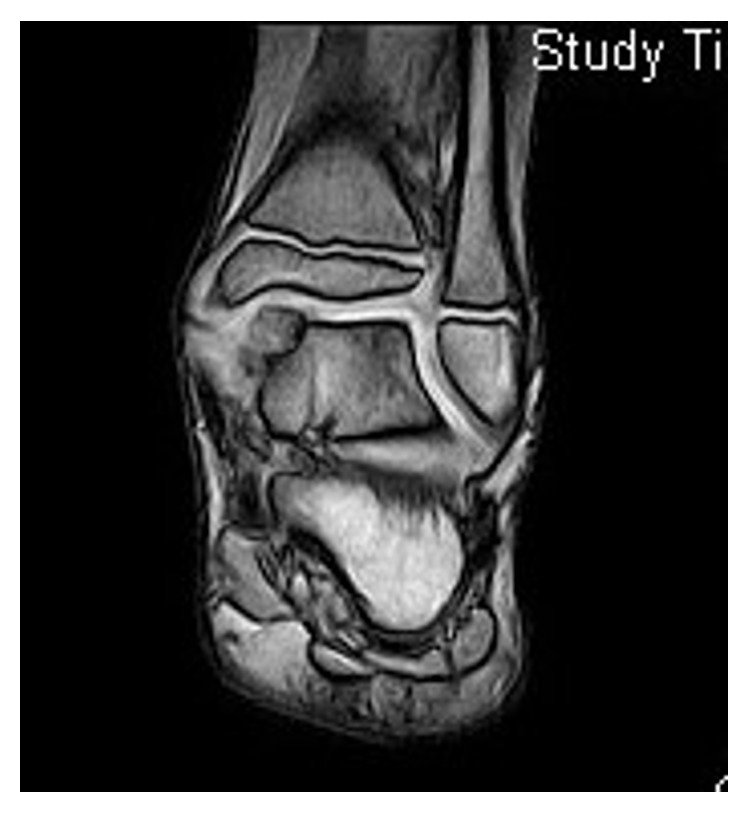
The MRI shows pedicled bone tumor arising from the talus.
